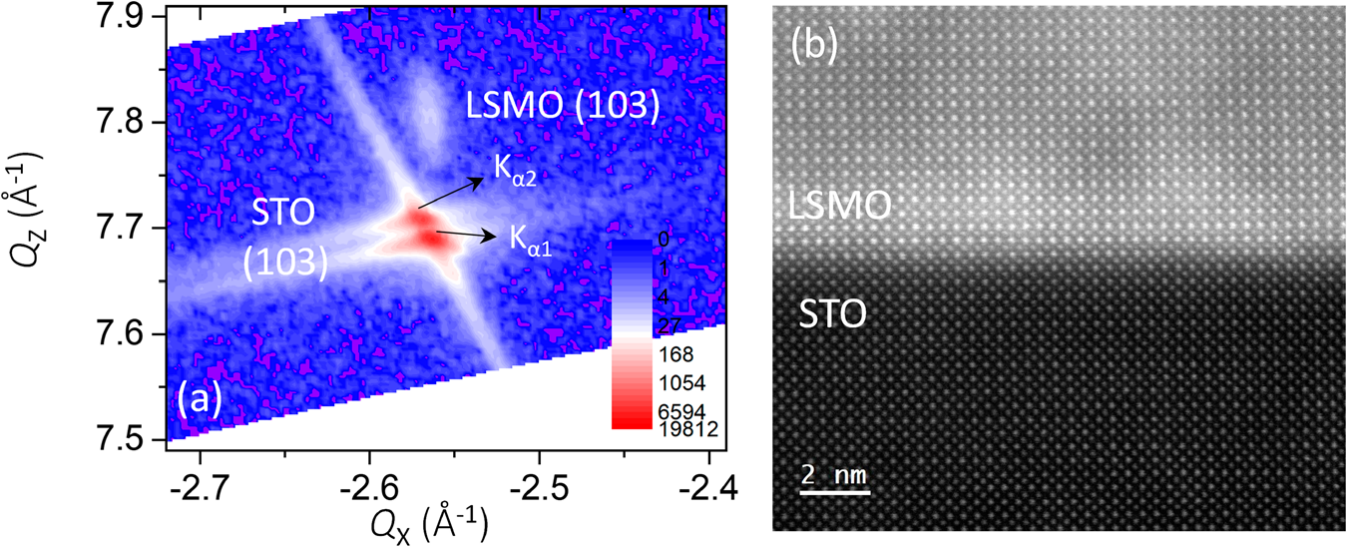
Structural characterization of the LSMO/STO interface. a Reciprocal space mapping around the asymmetric (103) Bragg reflection of 20 nm La_0.7_Sr_0.3_MnO_3_ grown on a SrTiO_3_(001) substrate. The arrows indicate the splitting of the substrate Bragg peak due to Cu-K_*α*1_ and Cu-K_*α*2_ radiation from the X-ray source. b An atomically resolved aberration-corrected STEM image of the cross-sectional view of the La_0.7_Sr_0.3_MnO_3_/SrTiO_3_ interface.
